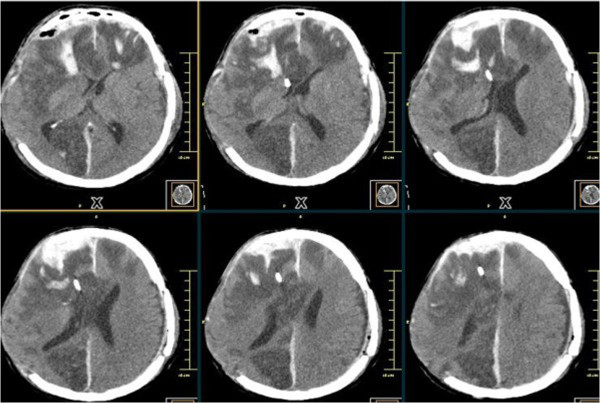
Computed tomography following drainage of extradural hematoma.
